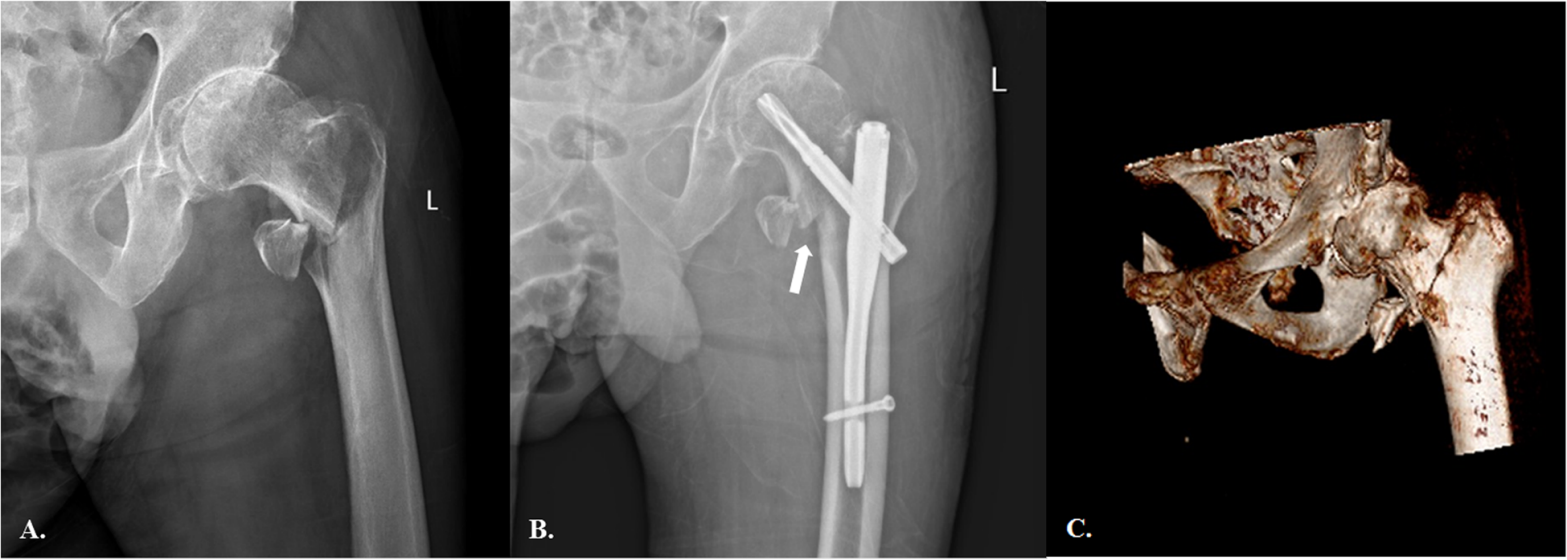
a The preoperative anteroposterior (AP) radiograph showing an apparently simple AO-31A1.3 (2018 version) pertrochanteric fracture. b Following fixation with PFNA, there was an obvious inferior gap at the basicervical region (white arrow) and moderate valgus deformity. The lucency around helix indicates a micro-motion and instability of the fracture-implantation complex. c However, the preoperative 3D-CT revealed that this case was a basicervical trochanteric fracture variant. On preoperative radiograph, the external rotation of the femoral shaft made the fracture line assessment and classification incorrectly
